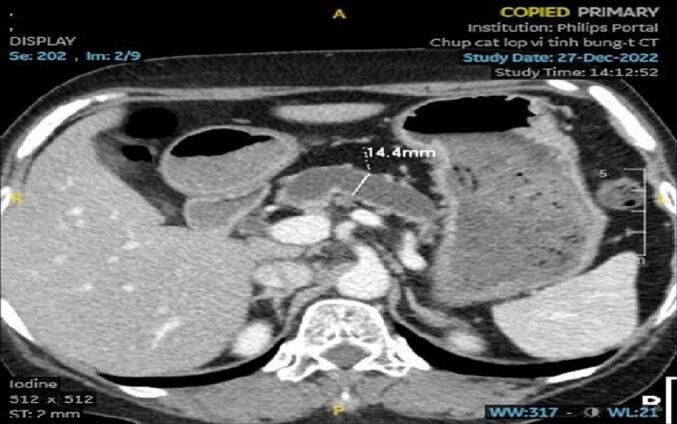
CT scan imaging: Dilated pancreatic duct.
